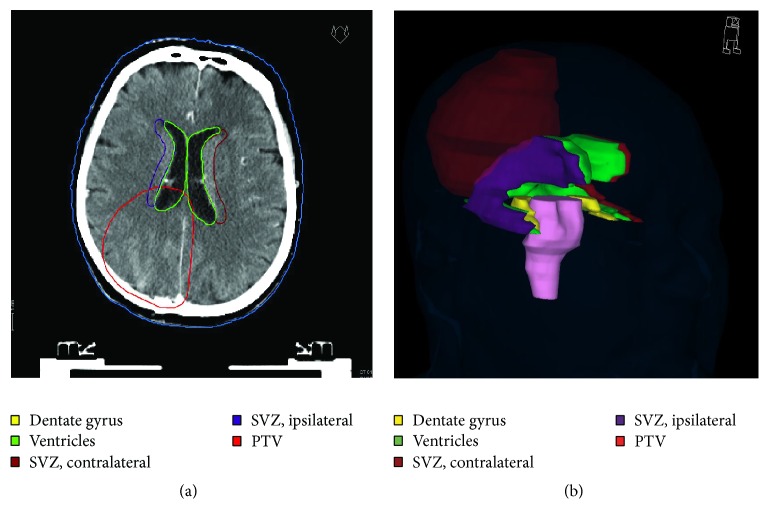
(a) Axial planning computed tomography with ventricles, subventricular zone (SVZ), and PTV. (b) 3D reconstruction including structures shown in the legend. The SVZ is defined as a 5 mm margin lateral to the lateral ventricle (purple and dark red). The brainstem (violet) is included for anatomical orienting.
